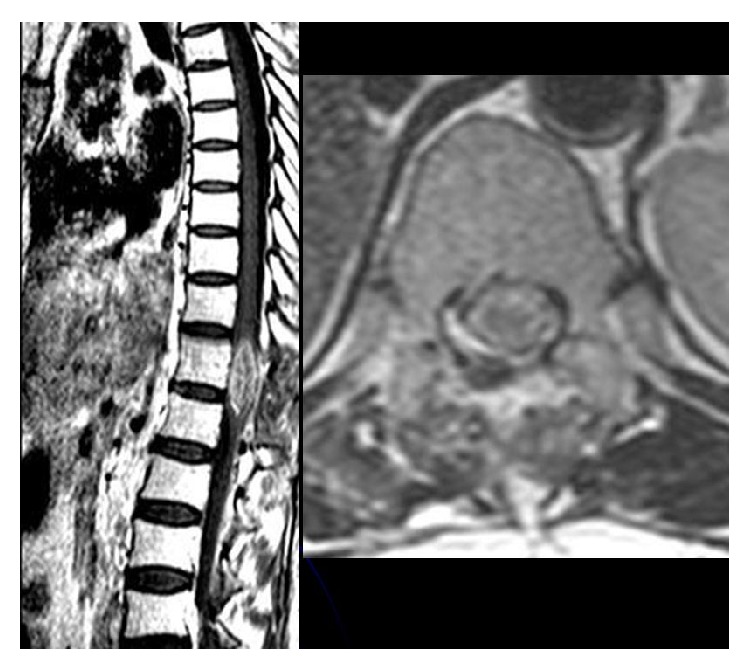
Postoperative spinal MRI showed not only evacuated inner part of the lesion but also remnant tumor adherent to neural tissue.
